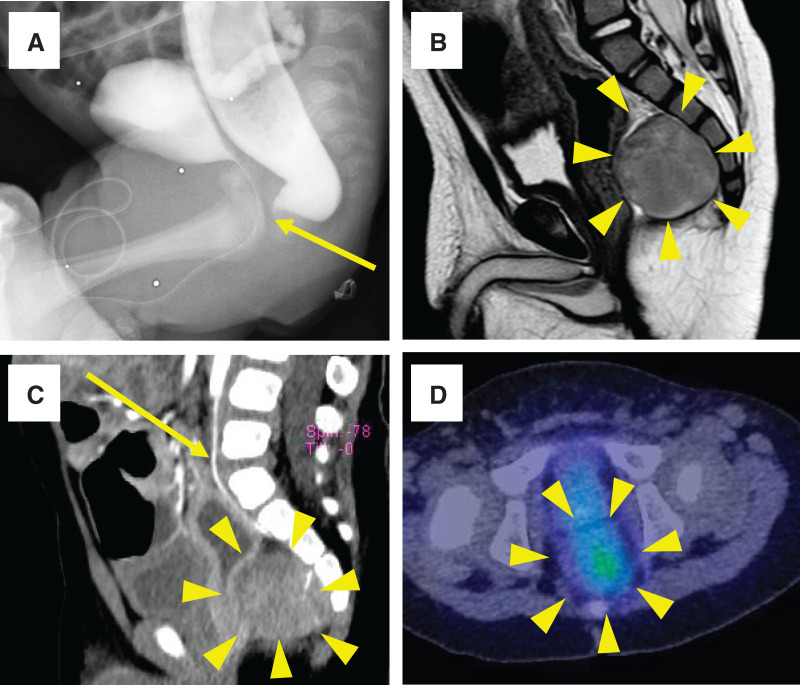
Imaging studies before surgery. (A) The imperforate anus was diagnosed as a rectobulbar urethral fistula (arrow) by voiding cystourethrography and colonorectography at 1 month of age. (B) Abdominopelvic MRI revealed a presacral tumor with a clear border and smooth surface. The tumor showed slightly high intensity on T2-weighted imaging (arrowheads). (C) Contrast-enhanced abdominopelvic CT showed that the tumor was a heterogeneously enhanced mass (arrowheads), and its feeding artery (arrow) was identified as the median sacral artery. (D) ^123^I-metaiodobenzylguanidine scintigraphy showed abnormal uptake in the intrapelvic presacral tumor (arrowheads).
